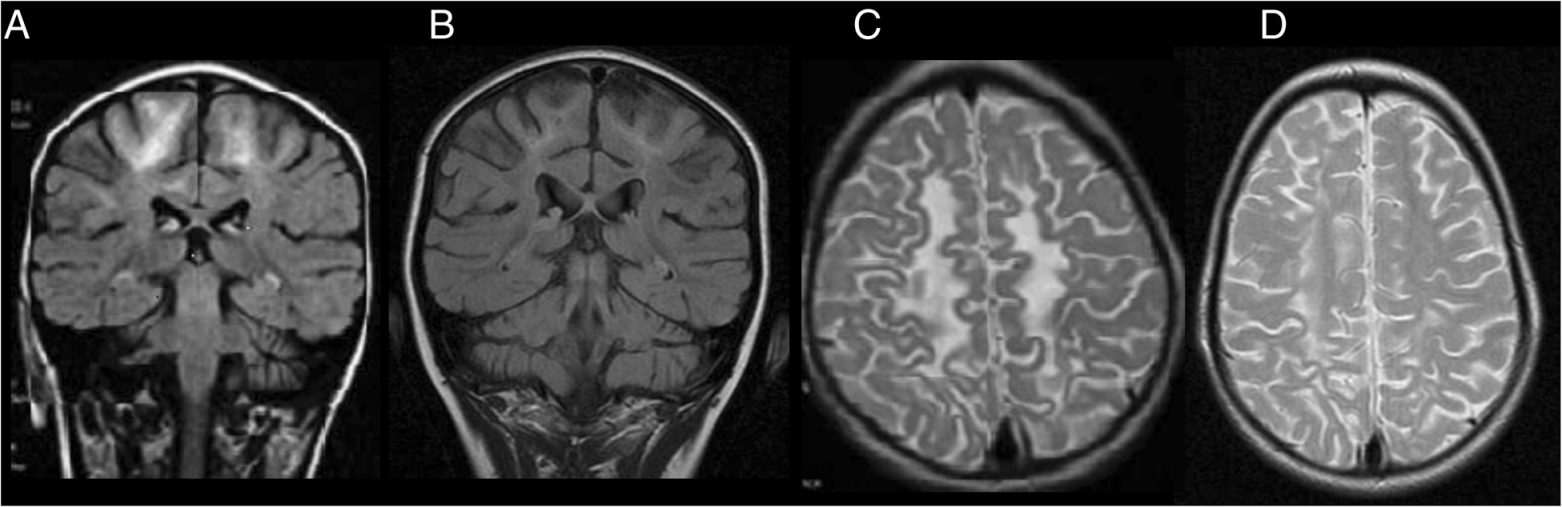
(Case 8): MRI coronal T2W. Abnormal high signal sparing the subcortical white matter with slightly periventricular location before folinic acid treatment were detected (A), improving after 2 years of folinic acid treatment (B). Abnormal high signal in the parietal white matter in MRI axial T2W was also observed before folinic acid treatment (C). Decreased of high signal after 2 years of treatment with near normal white matter signal (D).
